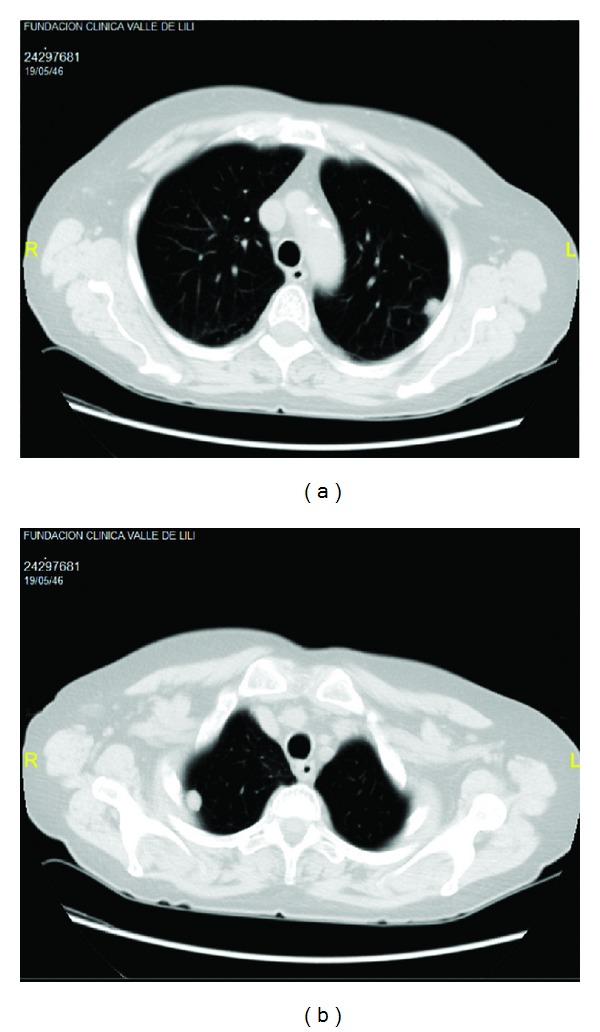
Pulmonary nodules seen on CT imaging of a patient with granulomatosis with polyangiitis.
